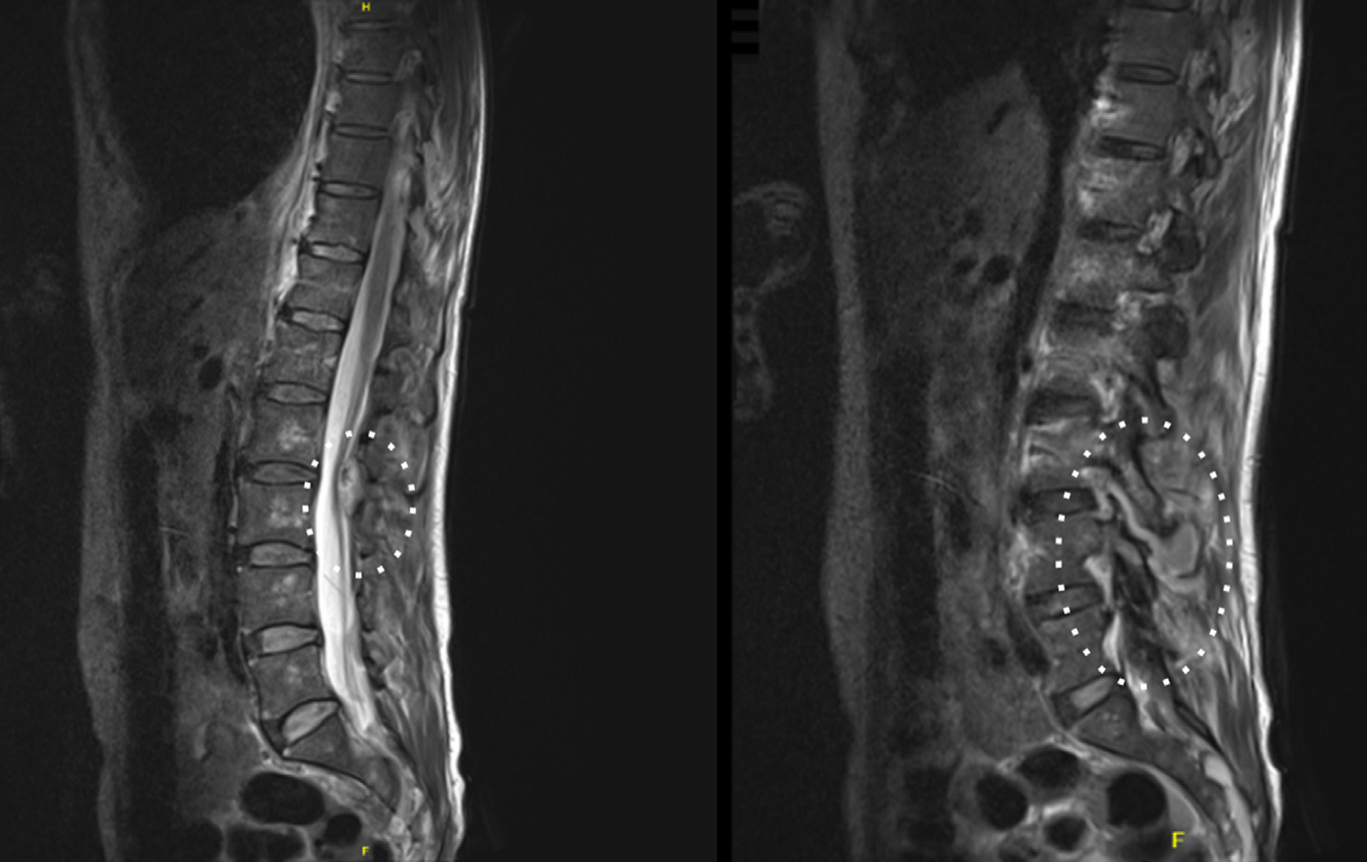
Facet joint septic arthritis and radiological spinal cord compression shown on the patient’s MRI spine scan taken in the acute phase of the disease.
